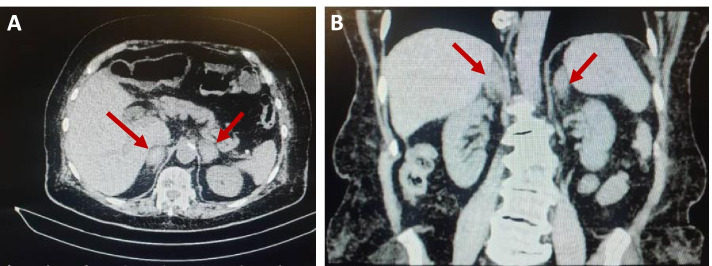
A computed tomography scan of the abdomen and pelvis of the second case shows bilateral adrenal masses with the same features on right and left sides with high density in non-contrast images. (A: axial view and B: Coronal view). The red arrows indicate adrenal hemorrhage
